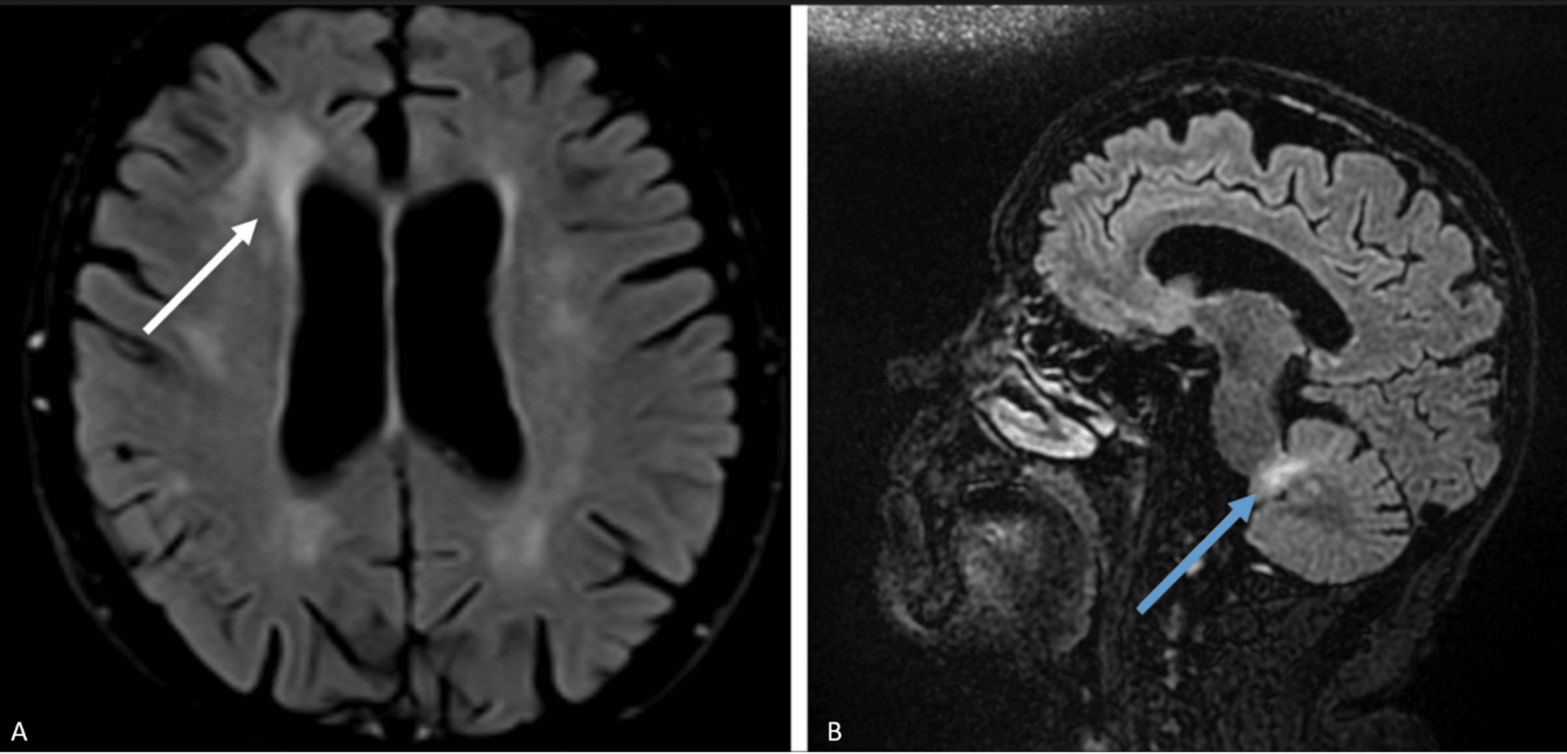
Brain MRI findings one month after corticosteroid therapy: Axial (A) and sagittal (B) FLAIR images show marked dilation of the lateral ventricles, diffuse periventricular and subcortical hyperintense lesions (white arrow), and a focal cerebellar hyperintense lesion (blue arrow). MRI, magnetic resonance imaging; FLAIR, fluid-attenuated inversion recovery
